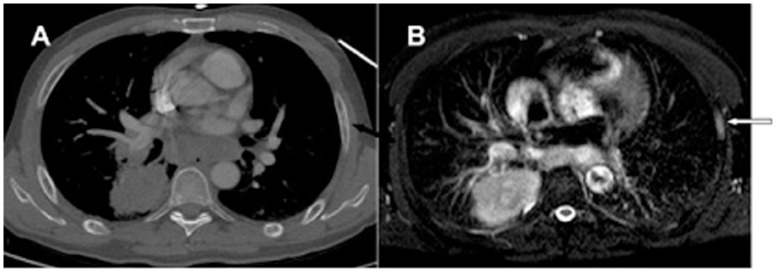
Minimal osteolytic changes: the initial manifestations of bone metastases and the primary stage of osteoblastic metastases. A. CT image showing a rib metastasis on the left, presenting as an intramedullary small patchy hyperdensity and a peripheral hypodense spot (black arrow). B. The lesion appears as an intramedullary area of slightly high signal on T2WI (FS) (white arrow).
